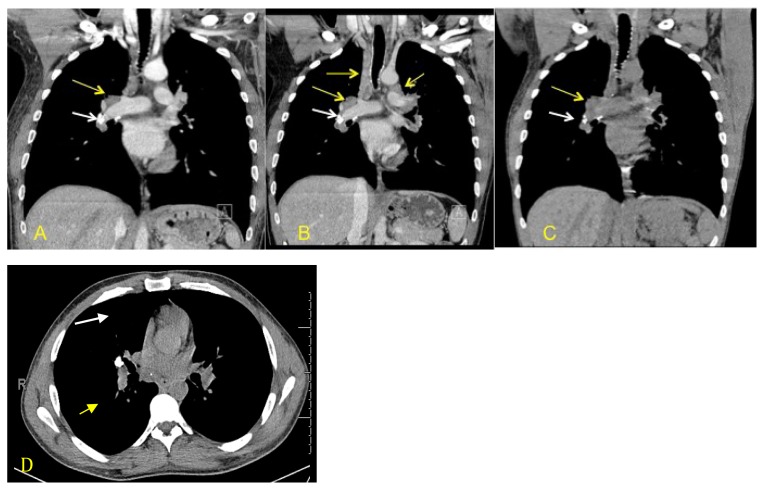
A) Coronal CT chest with contrast in 2013 demonstrated conspicuous right paratracheal and hilar lymph nodes. B) A 2014 scan demonstrated increased size of the right paratracheal and hilar lymph nodes (yellow arrows). C) Coronal CT chest without contrast in 2015 demonstrated slight increased size of the right hilar lymph nodes. D) Axial CT chest without in 2015 demonstrated calcified lymph nodes and right hilar lymphadenopathy. (White arrows point to calcified granulomas, indicative of a remote infection.) Endobronchial bronchoscopy fine needle aspiration in 2014 demonstrated non-caseating granulomas.
